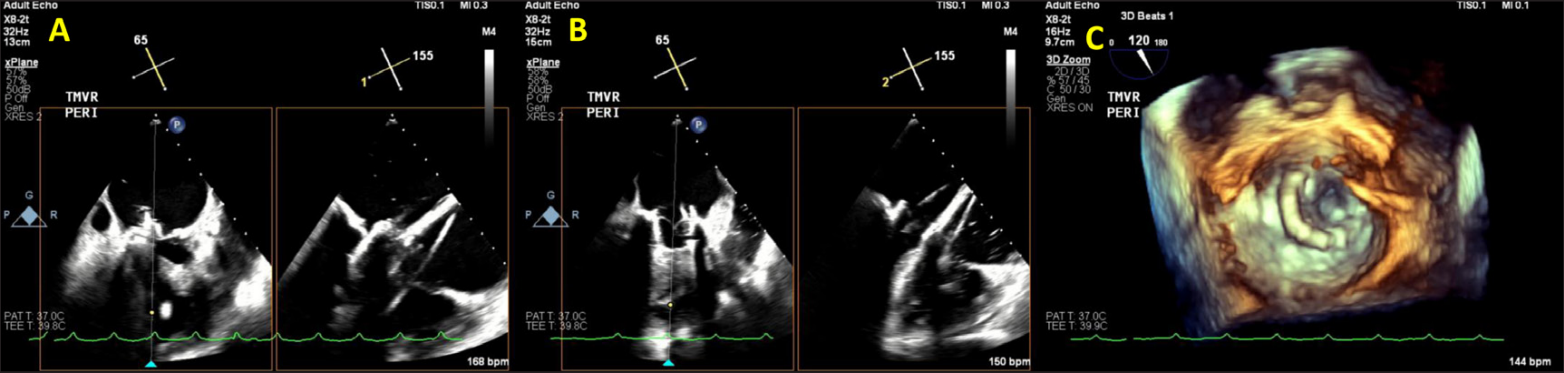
Transesophageal echocardiogram showing (A) transcatheter mitral valve implantation (TMVR) implantation. (B) Immediately after TMVR implantation, normal leaflet motion is noted. (C) 3D reconstruction demonstrating normal prosthesis leaflet motion. See Figure 7 A videoclip at https://youtu.be/ksmMxnu-oSk, Figure 7 B videoclip at https://youtu.be/r8dTvavy0jY and Figure 7 C videoclip at https://youtu.be/Yp4q9dn_os8.
